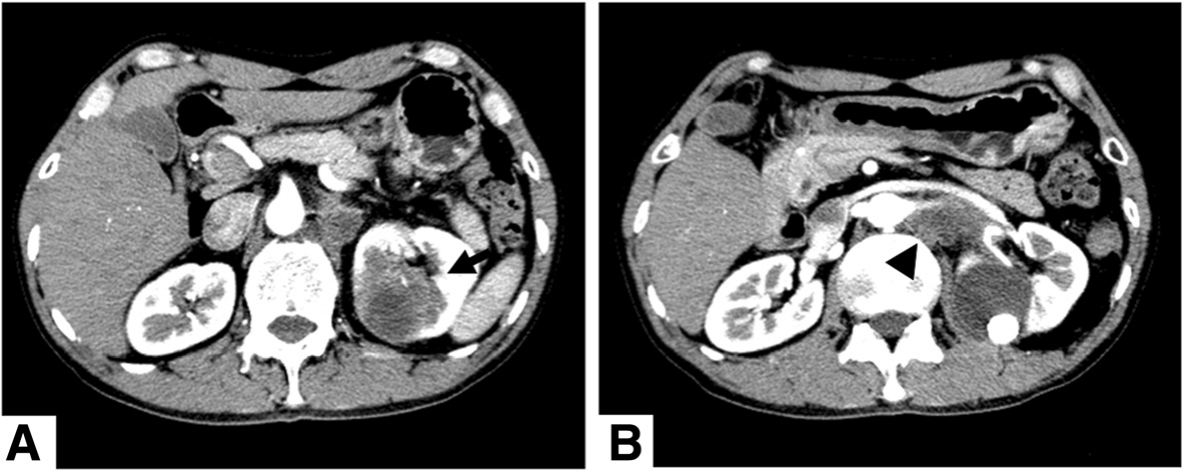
CT showed an irregular-shaped homogeneous mass (arrow) derived from the cyst and enveloped the renal pedicle
